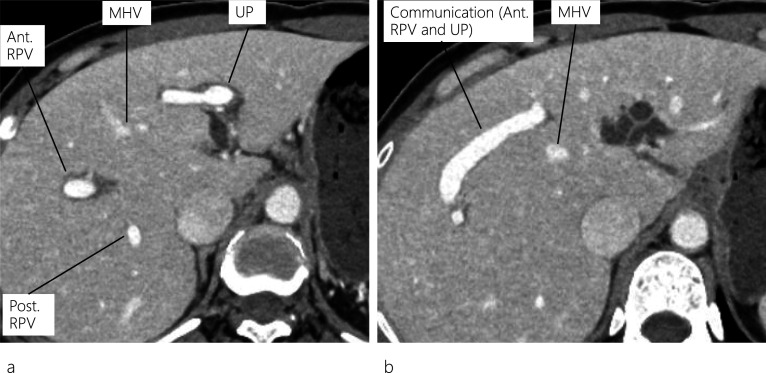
Enhanced CT images. Enhanced CT scan reveals a dilated IHBD in the left lobe and a communication between the right portal vein and umbilical portion (a, b). CT: computed tomography; IHBD: intrahepatic bile duct; MHV: middle hepatic vein; Ant. RPV: anterior right portal vein; Post. RPV: posterior right portal vein, UP: umbilical portion
